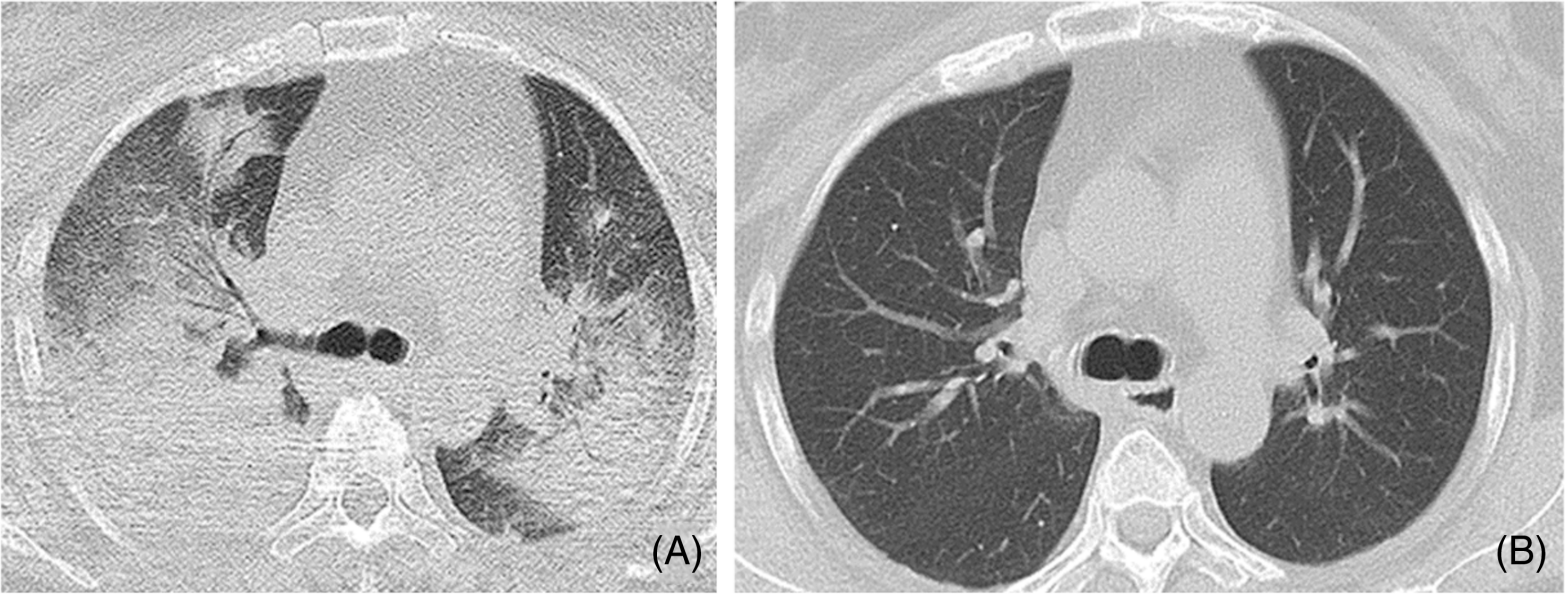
A CT scan (A) when she first came to our hospital, and (B) 4 months later after she started AMPC/SBT.
